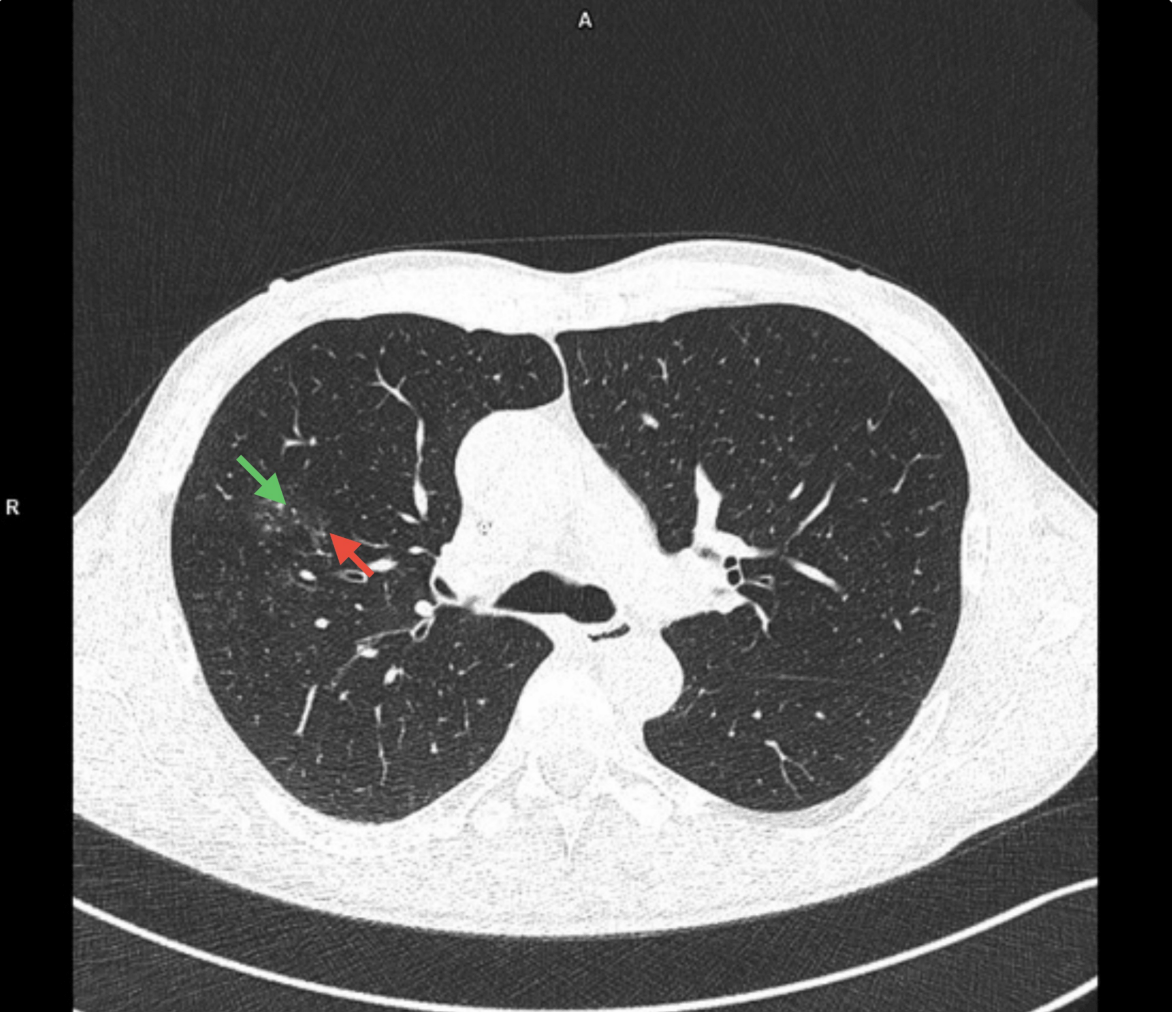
High-resolution CT of lungs High-resolution CT revealed peribronchial ground-glass opacities (green arrow) and tree-in-bud sign (red arrow) in second and third right lung segments.
